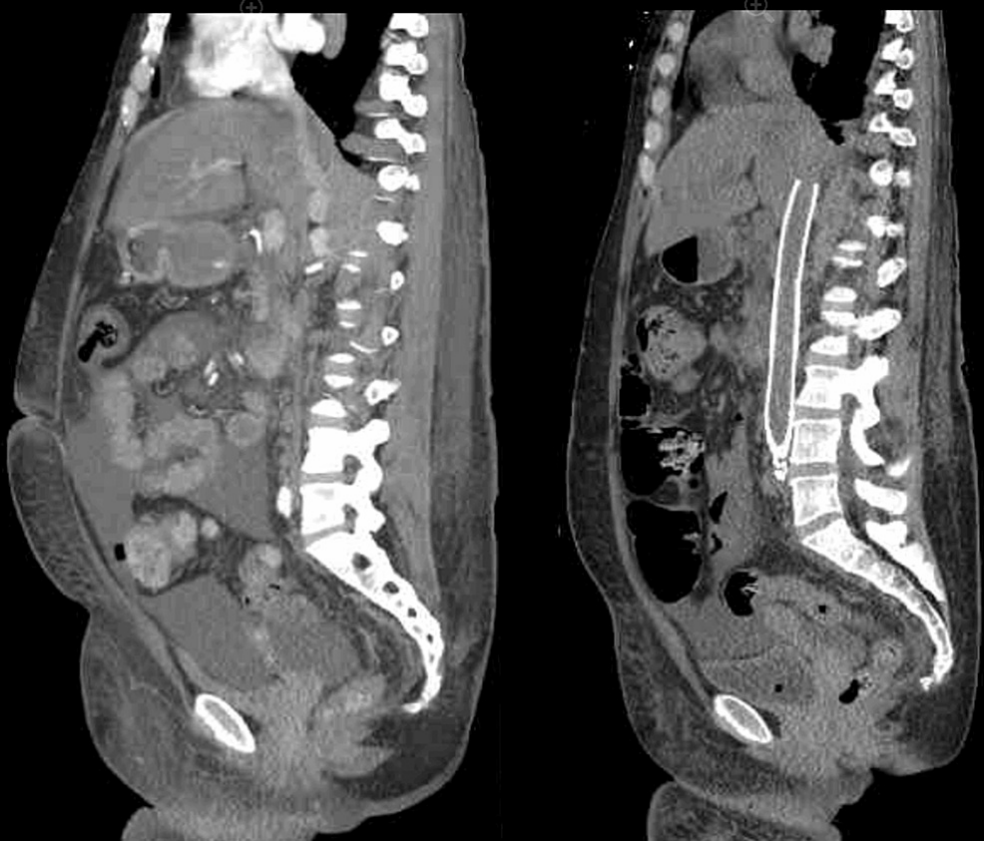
Sagittal abdominal/pelvis CT pre- and post-angioplasty Presents a sagittal image of the abdomen/pelvis, comparing pre-angioplasty (left, CTA) and post-angioplasty (right, CT without contrast). Following angioplasty, the CT image on the right demonstrates a notable reduction in anasarca and the volume of ascites. CTA: computed tomographic angiography; CT: computed tomography
